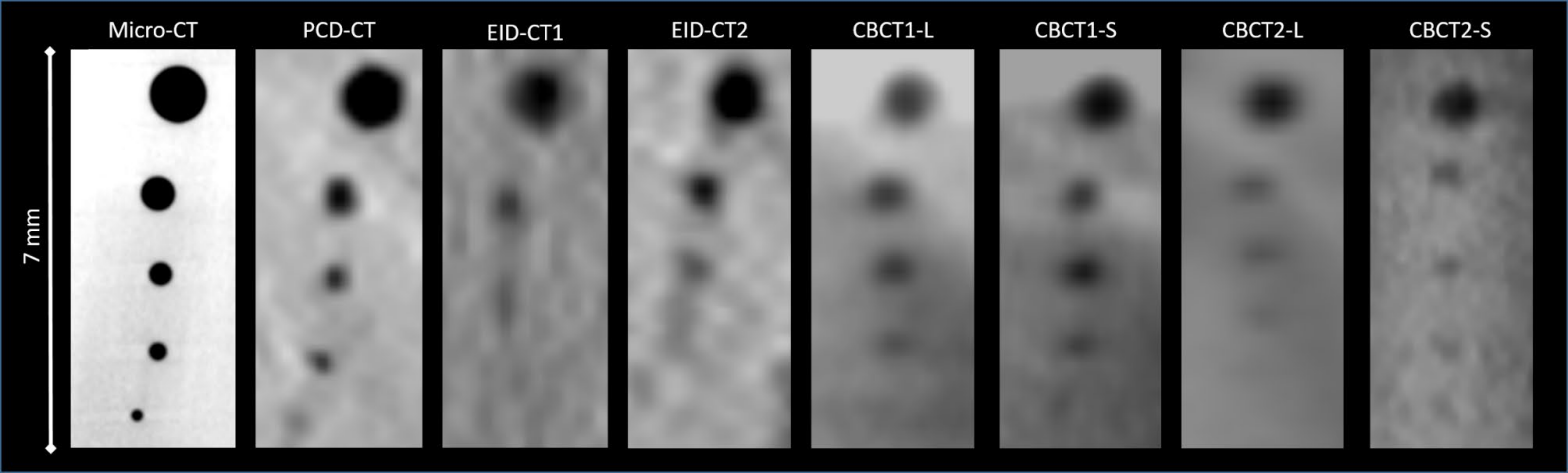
Exemplary images of the artificial accessory canals in all scanners. Analogous slices tangentially through the direction of the artificial accessory canals in the same tooth in all tested imaging systems (L for large FOV and S for small FOV). For visualization of the size, the white line on the right represents a distance of 7 mm. *PCD-CT* photon-counting CT, *EID-CT* third-generation energy-integrating detector CT, *CBCT* cone beam CT, *HU* hounsfield units.
